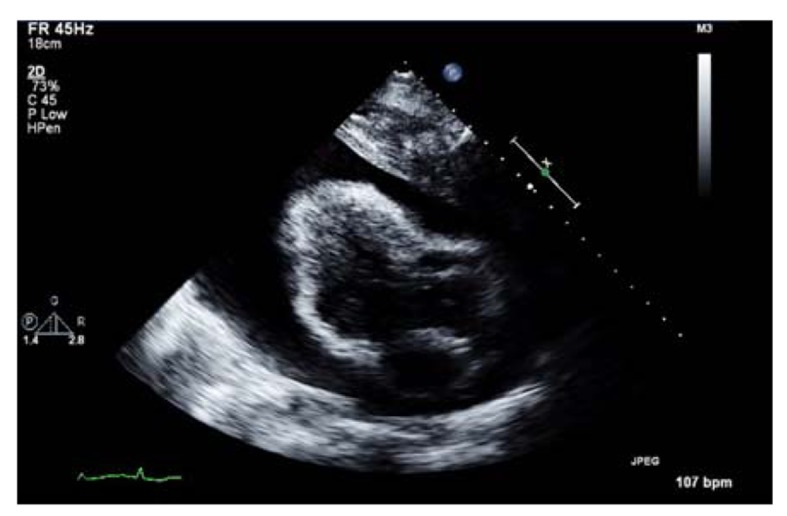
Transthoracic echocardiogram showing massive pericardial effusion.
